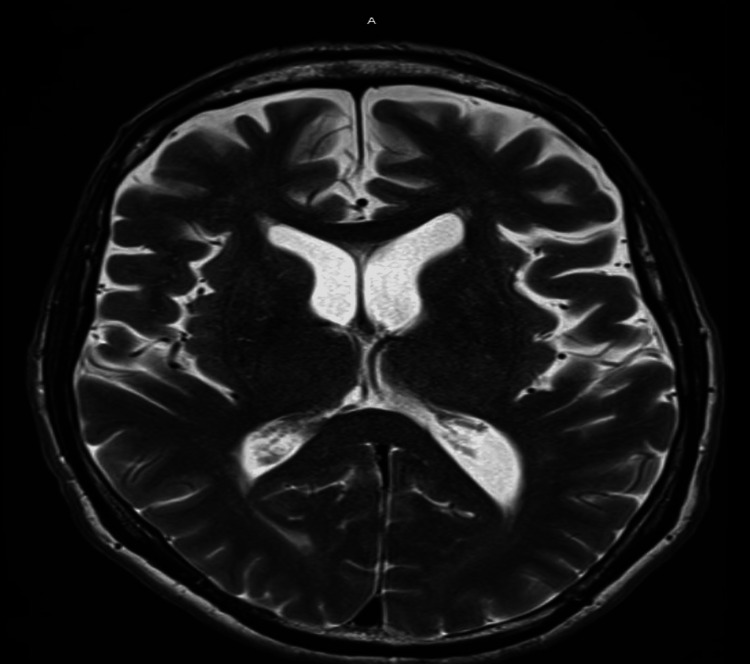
MRI brain showing normal findings. MRI: magnetic resonance imaging
